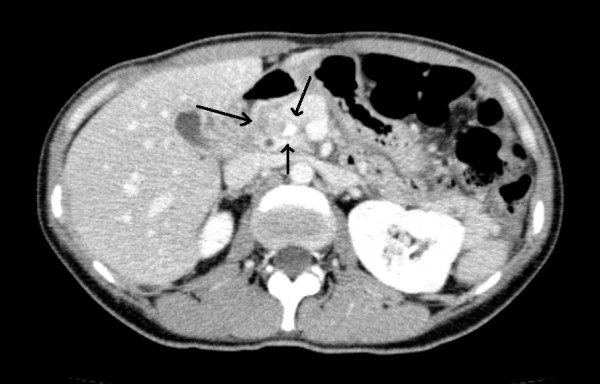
A computed tomography scan after tube exchange revealed remarkable improvement of the injured pancreatic parenchyma and resolution of the peripancreatic fluid collection.
